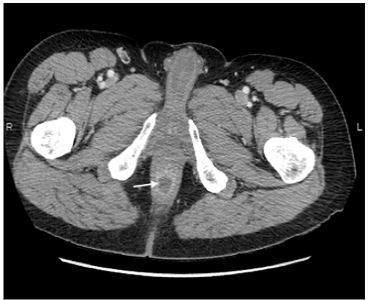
Axial computed tomography shows the intersphincteric abscess measuring 2.0 × 1.8 centimeters posterior to the anal canal (arrow).
